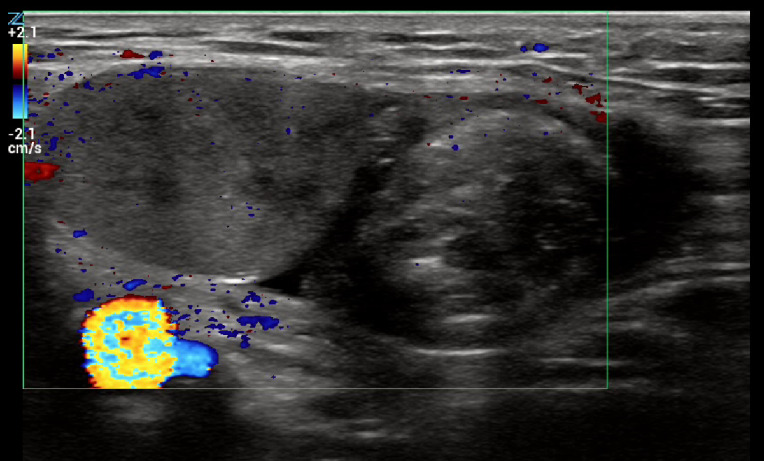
Color Doppler image with linear transducer. The Doppler gate is placed over a blood vessel and shows peaked, high amplitude flow.
